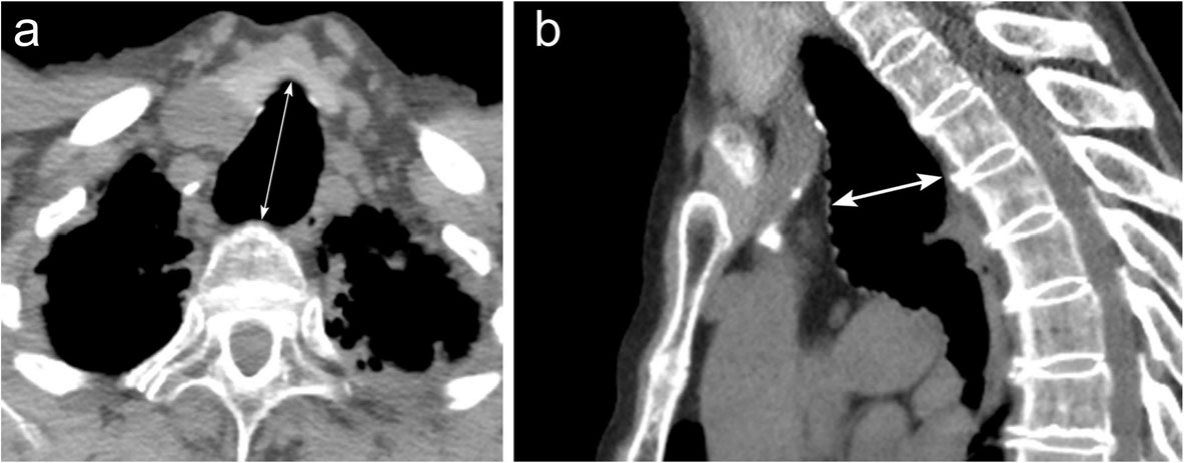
Tracheobronchomegaly. Axial (a) and sagittal (b) noncontrast CT images of the thoracic inlet demonstrating dilatation of the trachea, measuring > 3 cm as can be seen in the setting of tracheobronchomegaly
